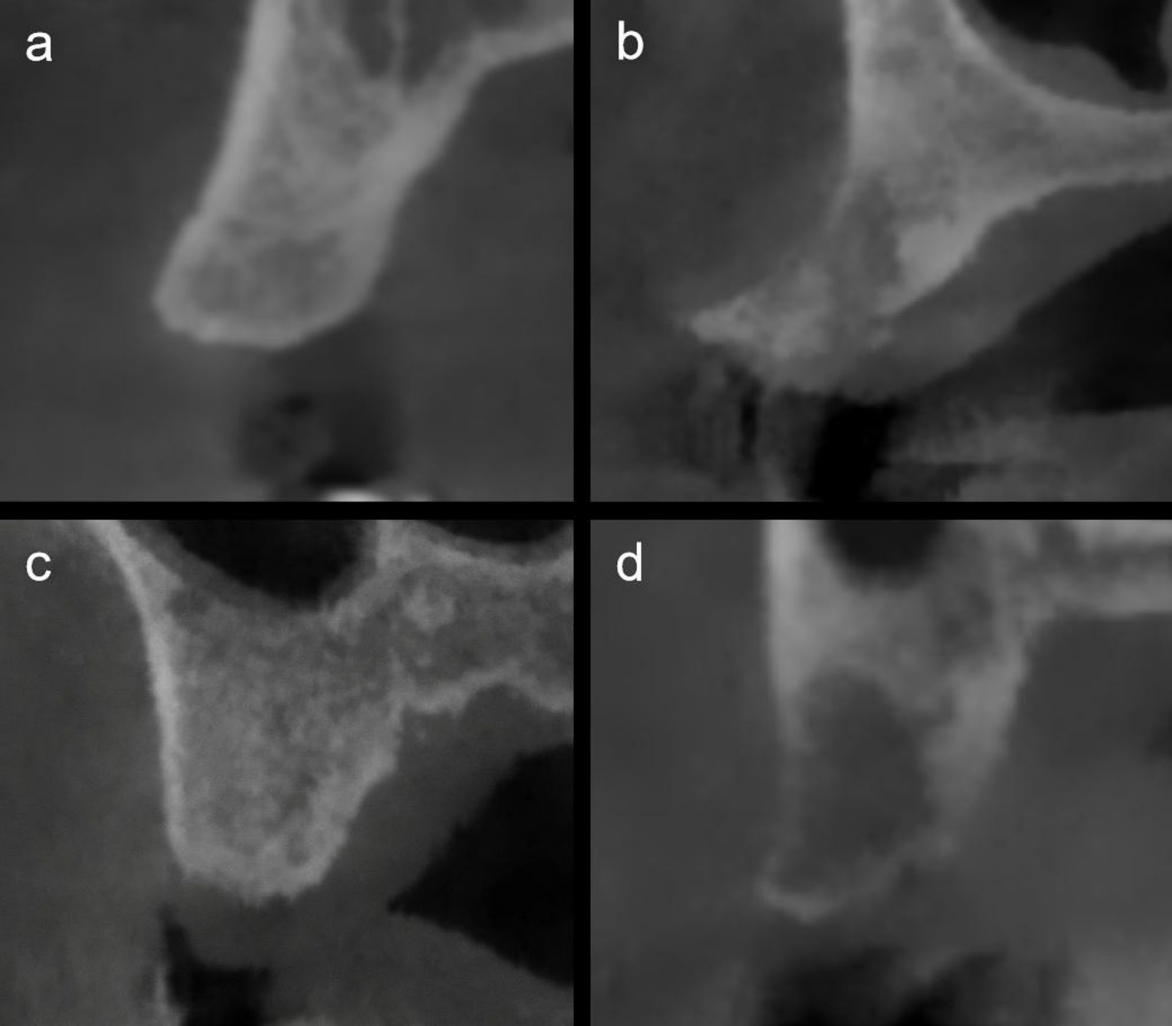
CBCT-based classification of alveolar bone morphology in the maxillary premolar region: a buccally projected-flat type; b buccally projected-concave type; c palatally retracted-flat type; d palatally retracted-concave type
